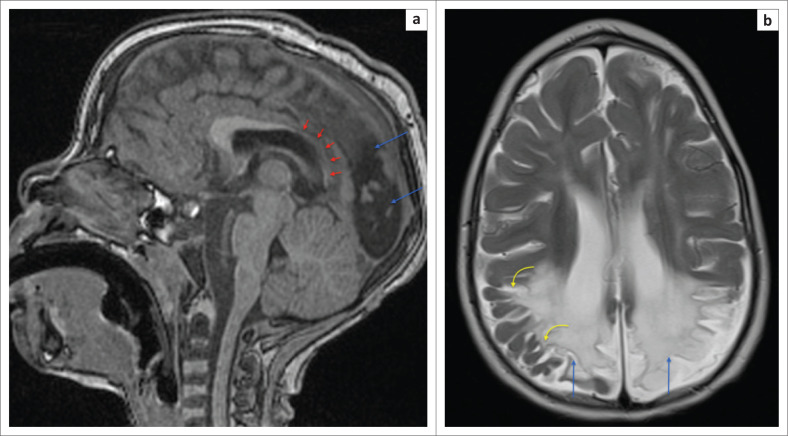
(a) Sagittal T1-weighted image at the midline of the calvarium demonstrating advanced spongiosis of the parietal and occipital lobes (blue arrows) associated with deafferentation thinning of the splenium and isthmus of the corpus callosum (red arrows). Note relatively normal calibre anterior body and genu of corpus callosum. (b) Axial T2-weighted image showing severe biparietal atrophy with ex vacuo dilatation of the lateral ventricles and evidence of ulegyria (yellow arrows). Note less marked changes in the frontal lobes.
